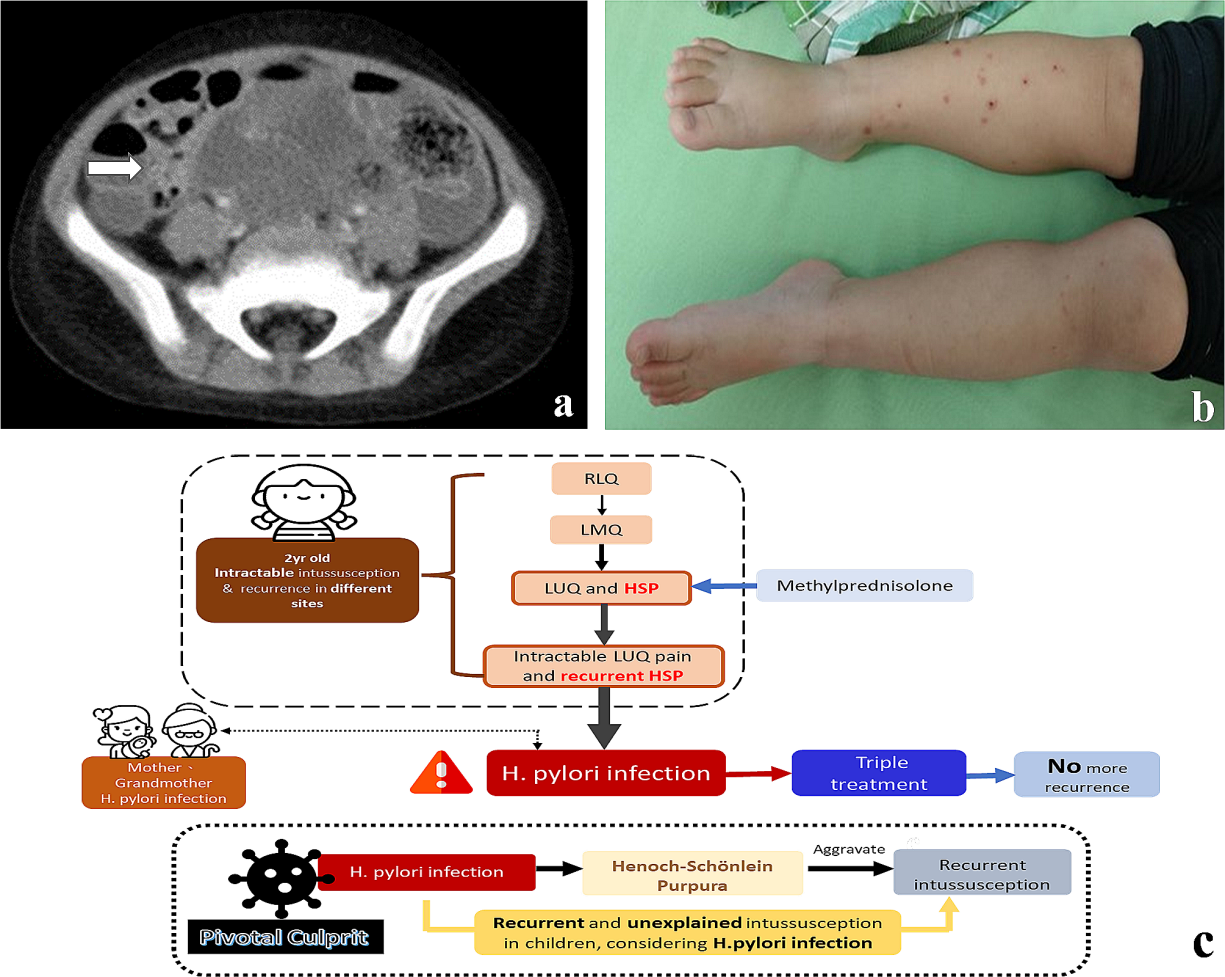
Exploring clinical manifestations and images of the index patient with recurrent intussusception. a. CT image showed enlarged mesenteric lymph nodes. b. Multiple purpuric macules and papules over lower limbs. c. Clinical role of H. pylori infection as a culprit in recalcitrant intussusception and HSP
